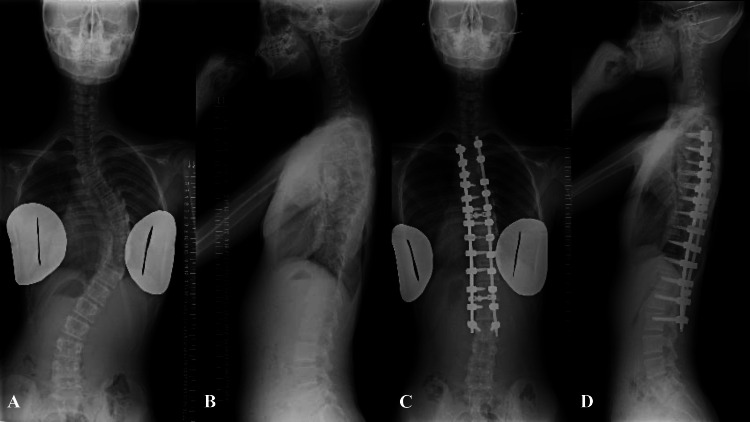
Case 2. Plain radiographs of the whole spine in a standing position. A: preoperative AP view, B: preoperative lateral view, C: postoperative AP view, and D: postoperative lateral view.
